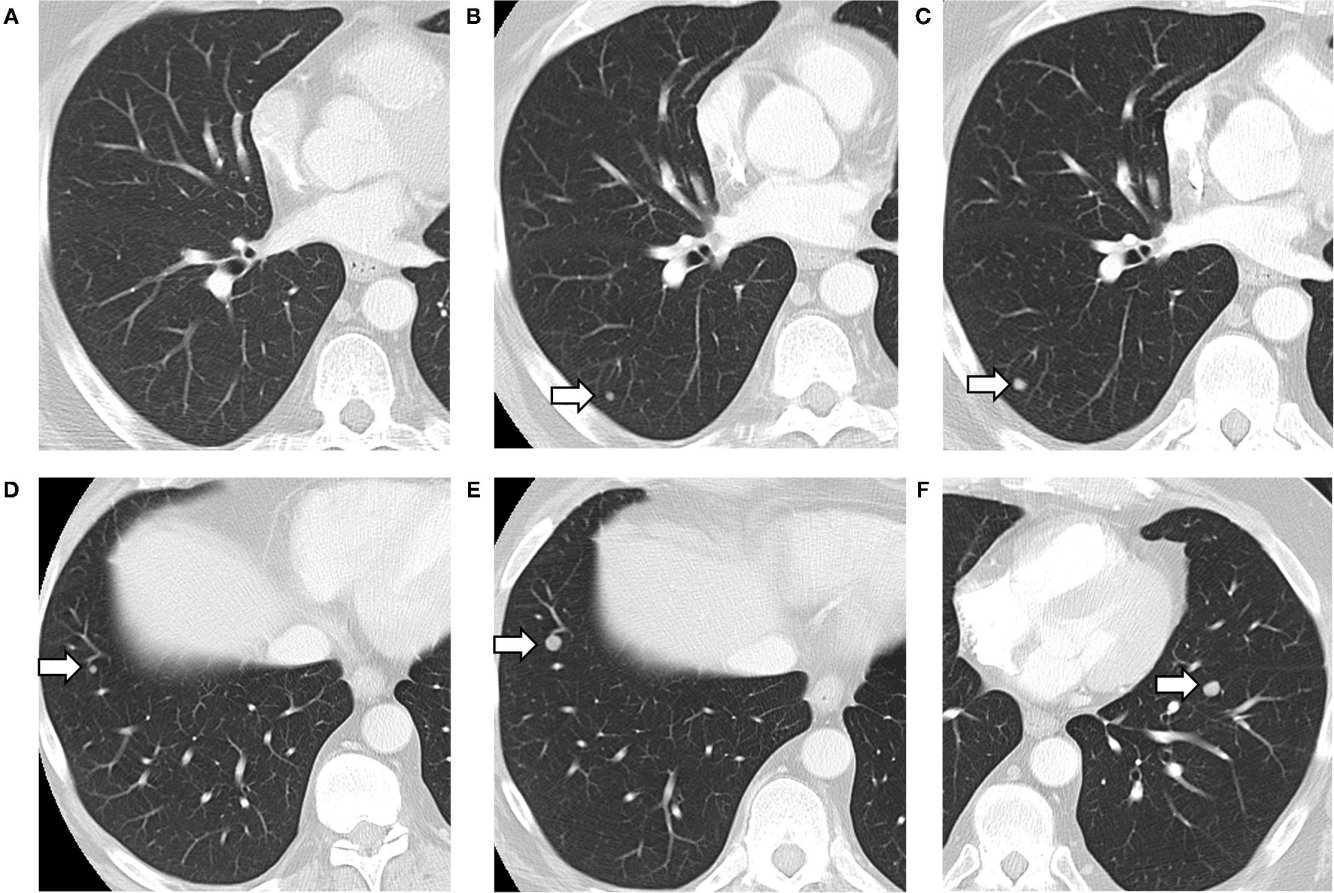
Lung metastases developing on serial CT scans (arrows). Axial images of the lung from three consecutive CT scans, showing the development of a lung nodule, in the posterior right lower lobe (A–C). A separate nodule in the anterior right lower lobe also grew between the second (D) and third CT scan (E). A third nodule appeared in the left lower lobe on the third scan only (F). The first CT was negative for metastasis (A), with the text in the “Lungs” section of the findings reading “No suspicious findings.” The model predicted correctly that there were no metastases described with 100% confidence. The second CT (B,D) was labeled as positive for metastases by the radiologist who had access to all three scans, but negative by the CNN (with a confidence of 99.60%) which only had reports for the first two. The third CT (C,E,F) was labeled as positive by both radiologists and the CNN (with 100% confidence).
